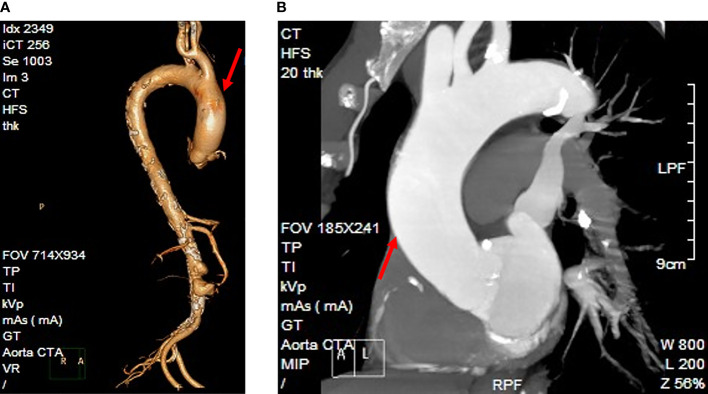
The patient’s diagnostic imaging. (A) Reconstruction of the CT angiography image. (B) CT angiography indicated an unruptured abdominal aortic aneurysm (arrowhead).
